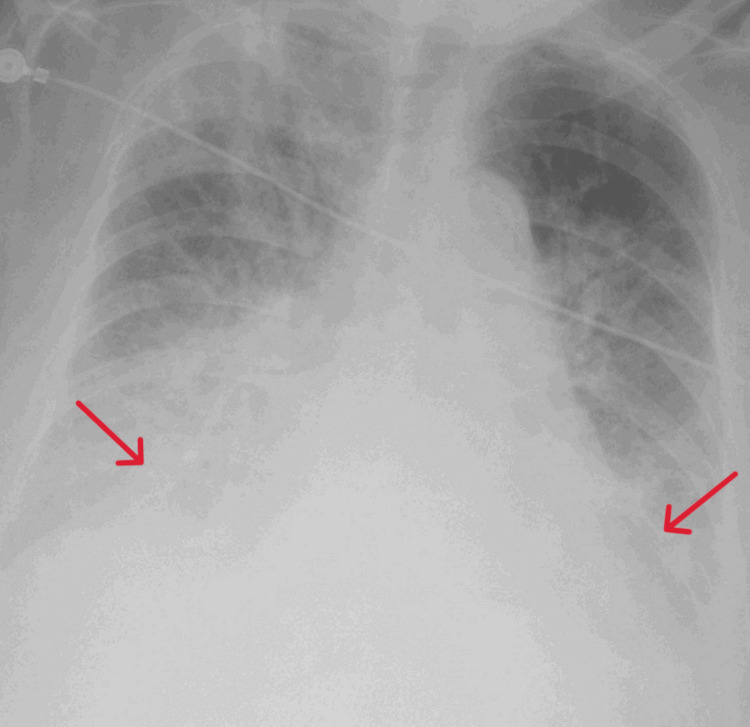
Chest X-ray demonstrated a prominent bilateral pulmonary vasculature and bilateral pleural effusions (arrows).
